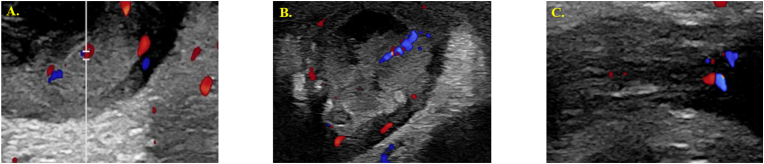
Ultrasound results on 1-Week Follow-up Visit. (A) Right Testicle, (B) Right Epididymal Head, (C) Right Inguinal Canal
